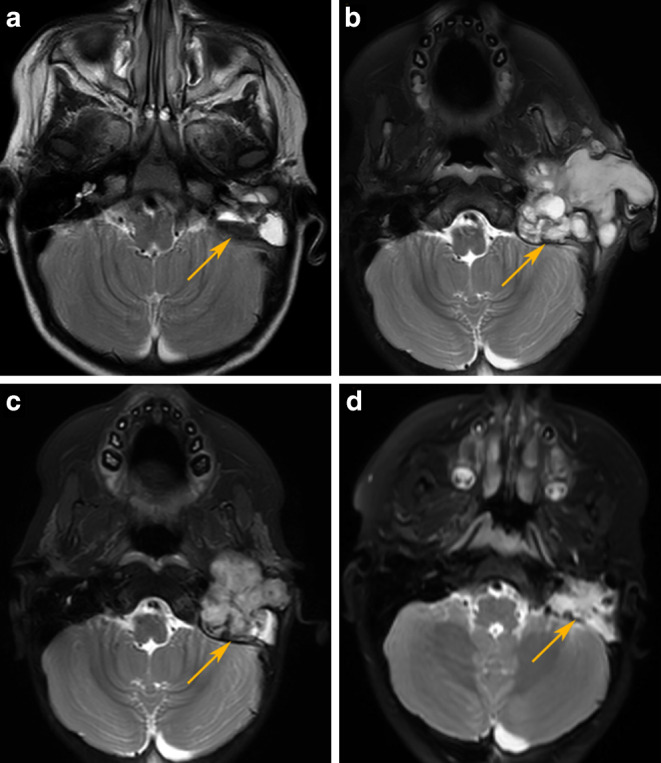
a–d Axial T2W images. a The initial MRI (June 2018) shows a cystic lesion in the left mastoid region with fluid–fluid level (arrow). At the first follow-up examination (b; July 2018) during active chemotherapy, in the T2 FS image, we can see 75% progression with the appearance of a solid tumor component as well. The next follow-ups (T2 FS images) show the tumor before radiation therapy (c; February 2019) and 4 years after radiation therapy (d; July 2023)
